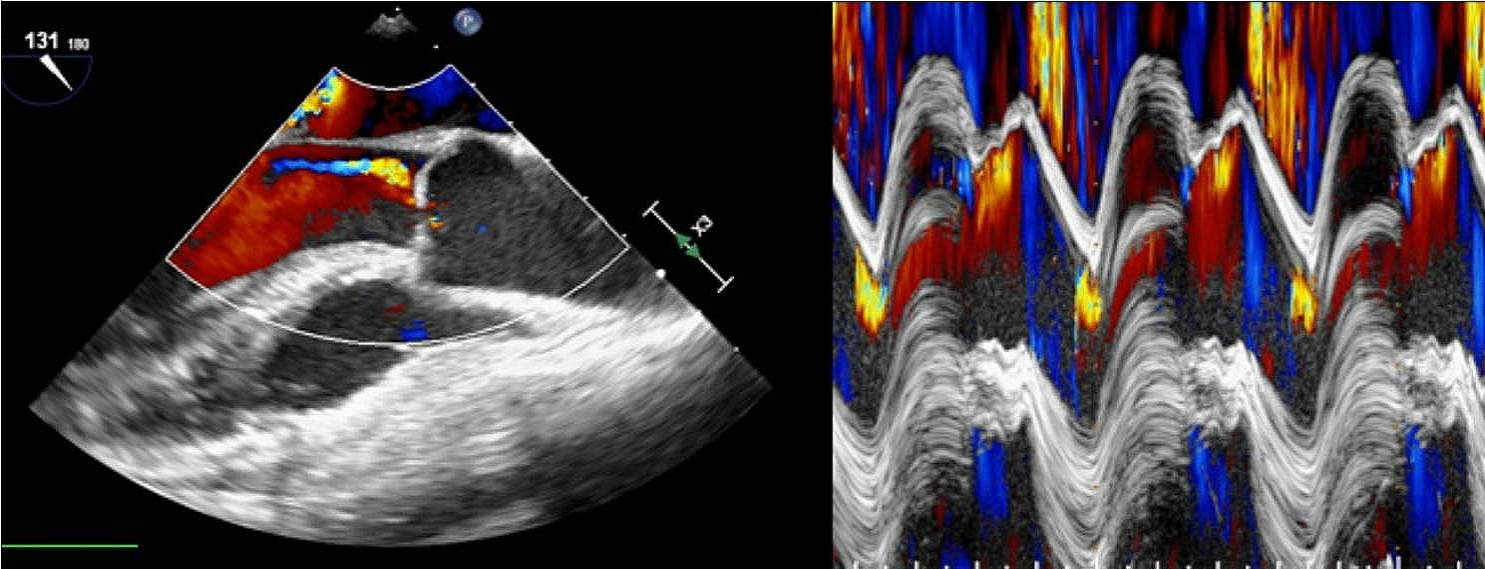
Color doppler 2D and color enhanced M-mode images from intraoperative transesophageal echocardiography with IABP in place
